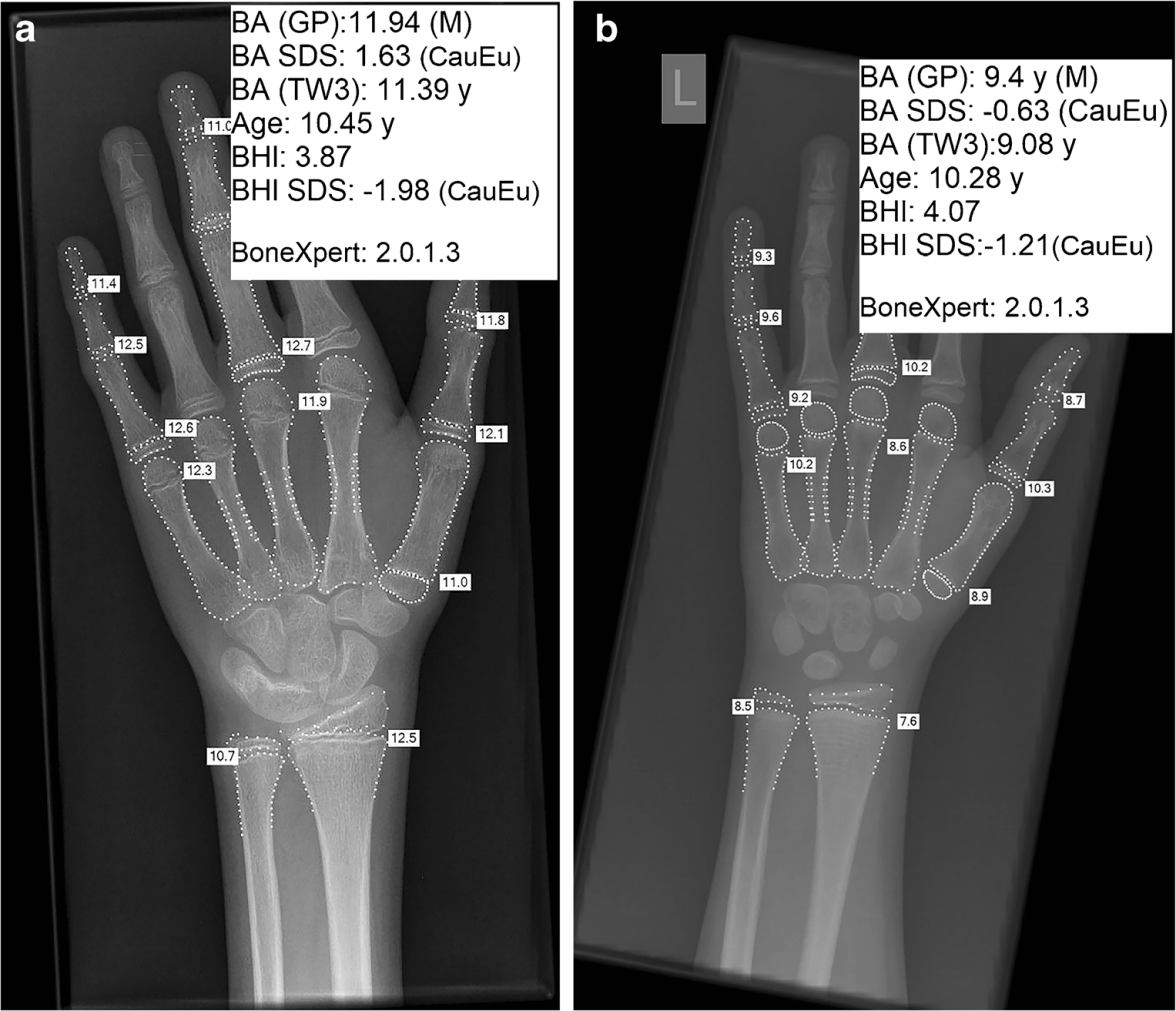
Left-hand radiographs in two 10-year-old Caucasian boys show relatively similar bone health index: (a) bisphosphonate naïve and (b) on bisphosphonate treatment. *BA (GP)* bone age using Greulich and Pyle’s atlas, *BHI* bone health index, *SDS* standard deviation score, *TW3* Tanner-Whitehouse 3
